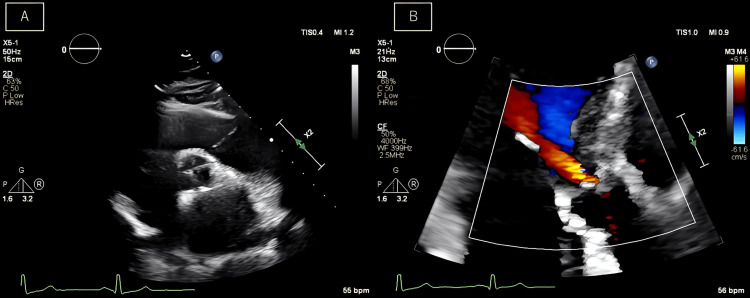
Preoperative transthoracic echocardiogram (A) Preoperative echocardiogram showing a quadricuspid aortic valve. (B) The aortic regurgitant jet is not vertical, but ubiquitously depicted toward the mitral cusp.
